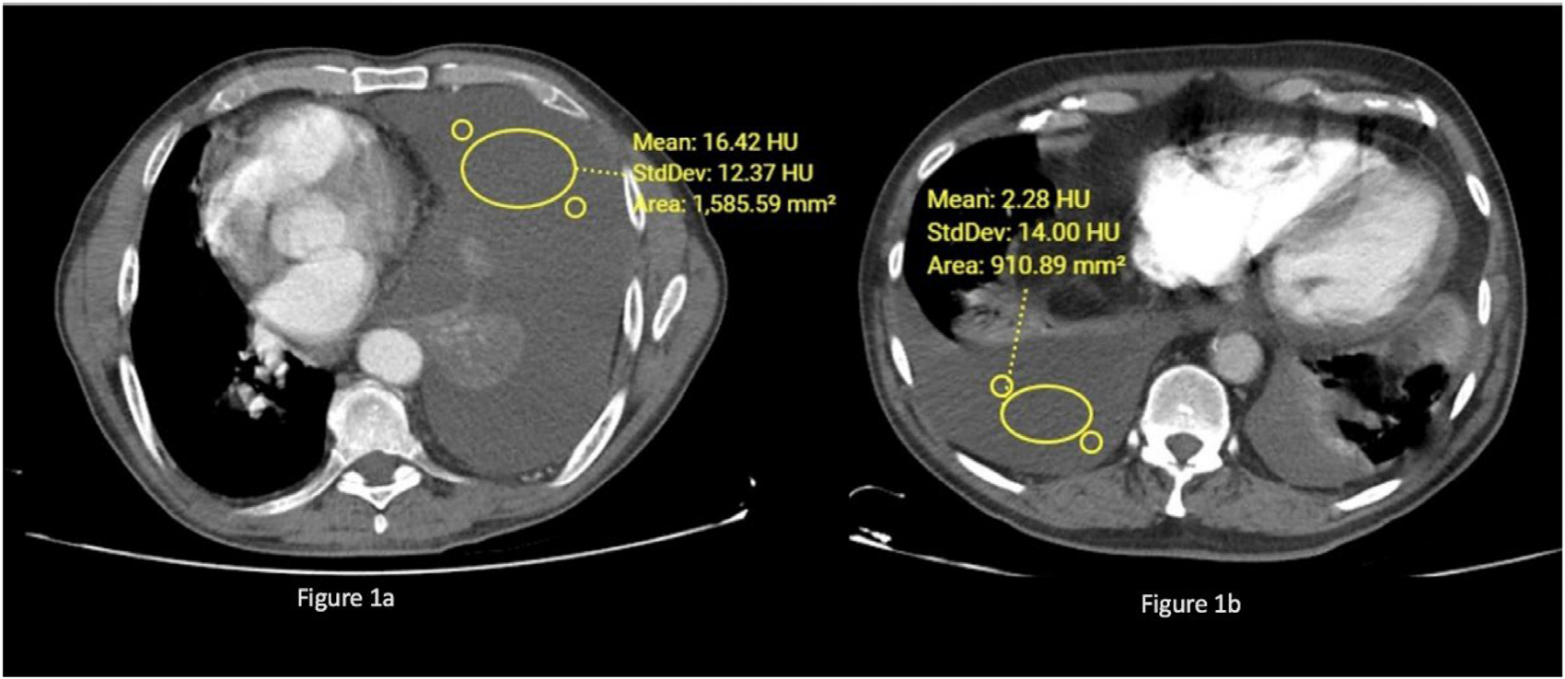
(a) The mean attenuation value of a unilateral pleural effusion due to lung adenocarcinoma in axial sections of contrast-enhanced thorax CT of a 63-year-old patient was measured as 16.42 HU. (b) The mean pleural fluid attenuation value of the bilateral pleural effusion of a 60-year-old patient with CHF was measured as 2.28 HU in axial sections of contrast-enhanced thorax CT.
